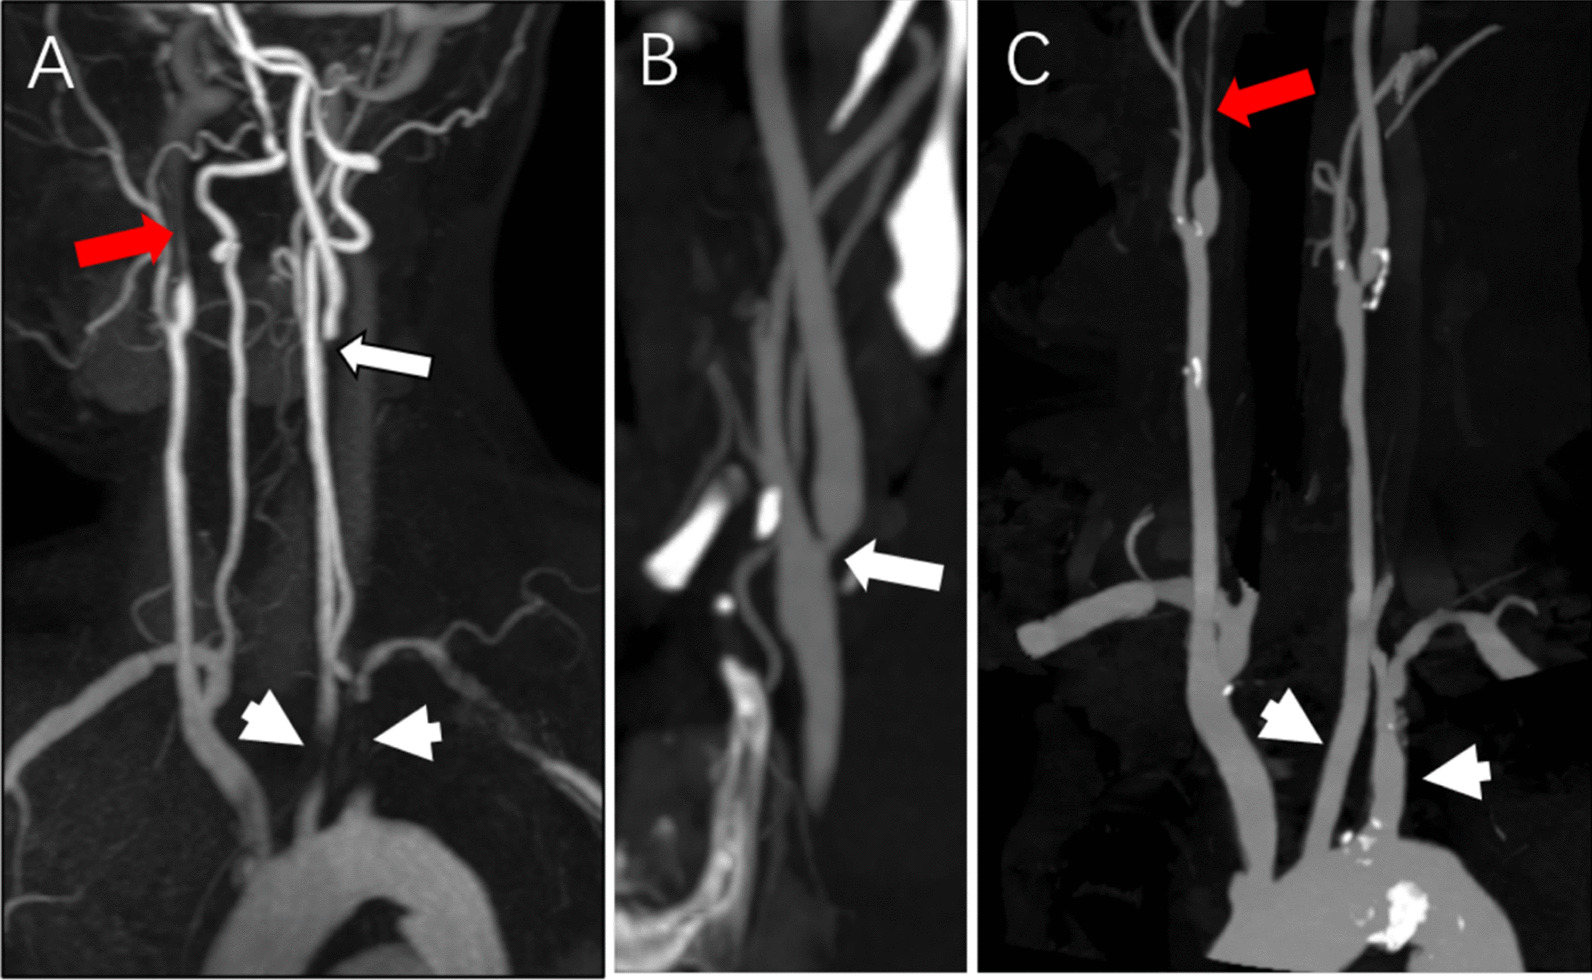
The same patient described in Fig. 6. In station I (head-neck region) of whole-body subtractionless CS-WBMRA MIP (A), an occlusion in the left internal carotid artery (long arrow) was detected but was graded as severely stenosed (> 90% stenosis) by carotid CTA (B, long arrow). In A, there seemed to be occlusions in the left common carotid artery and the left subclavian artery (short arrows), which were considered to be normal by CTA (short arrows in C). The distal part of the right internal carotid artery had severe stenosis (red arrow in A), causing a slight enlargement of the proximal part, which was confirmed by carotid CTA (red arrow in C)
